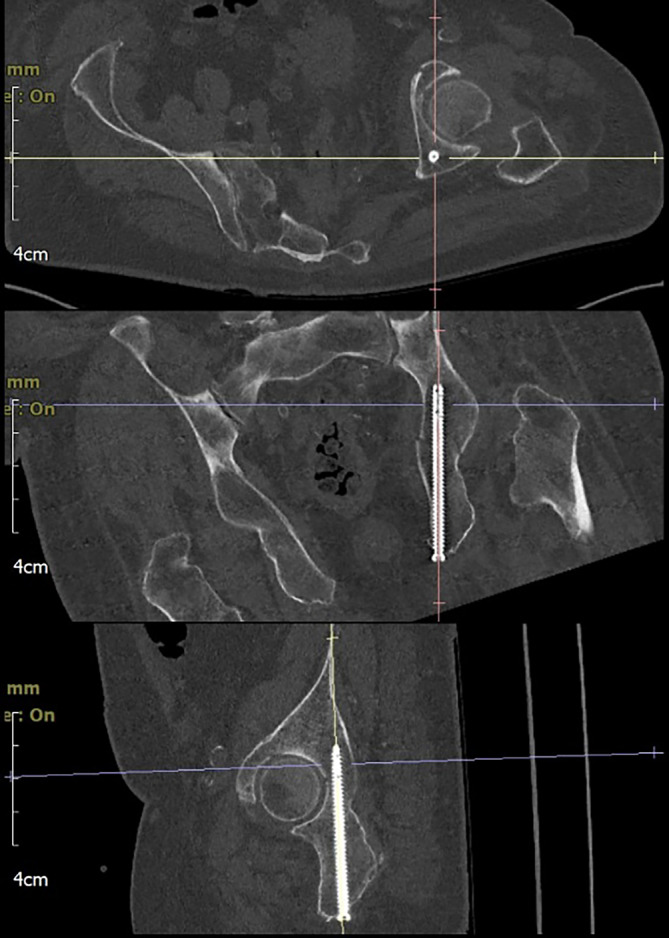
Postoperative multiplanar CT of the retrograde posterior column acetabular screw
